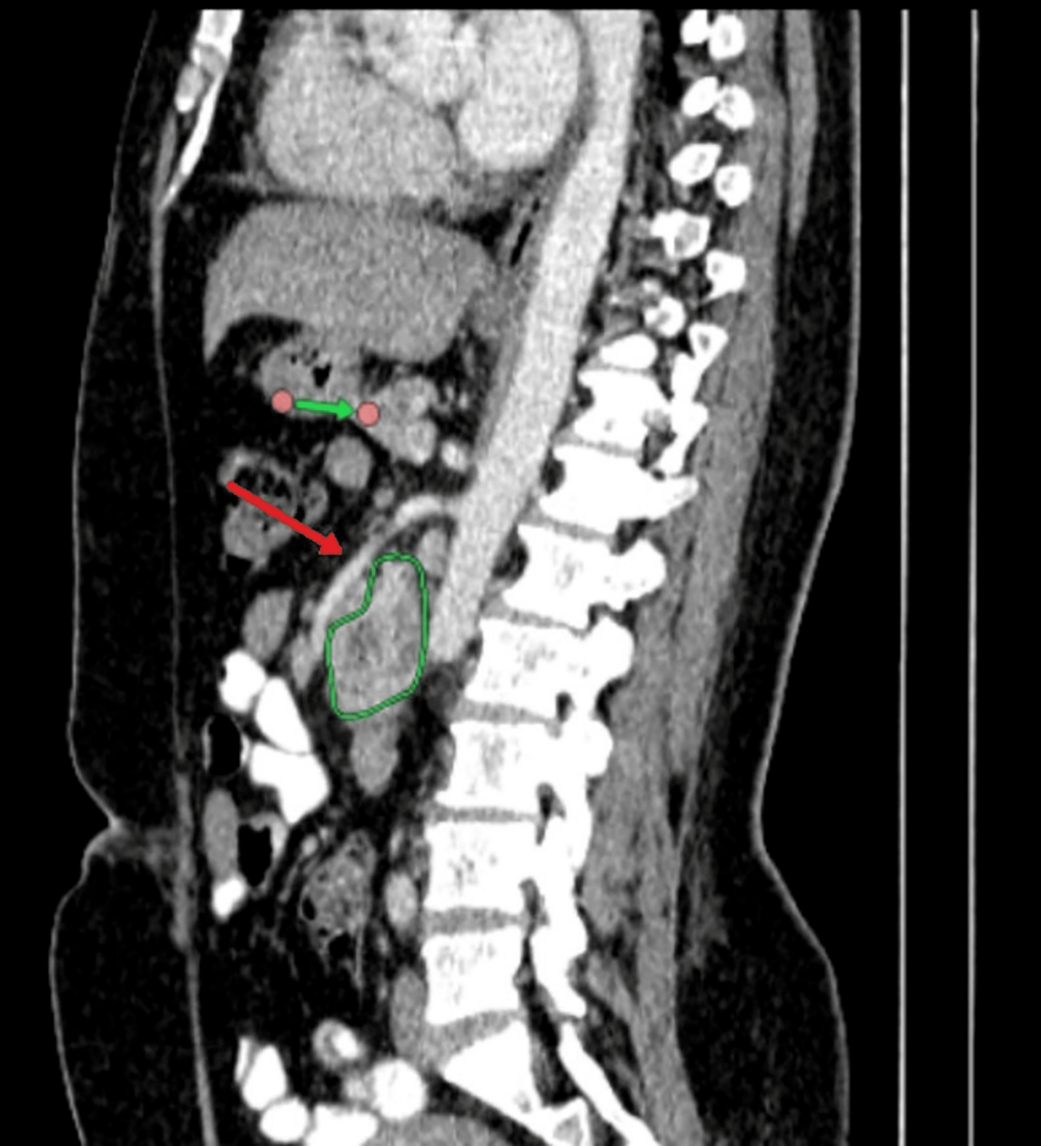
Sagittal view of the abdomen CT scan shows a well-defined mass in the duodenum (outlined in green) with the red arrow indicating its proximity to nearby vascular structures. This mass is consistent with a GIST. GIST: Gastrointestinal stromal tumor
